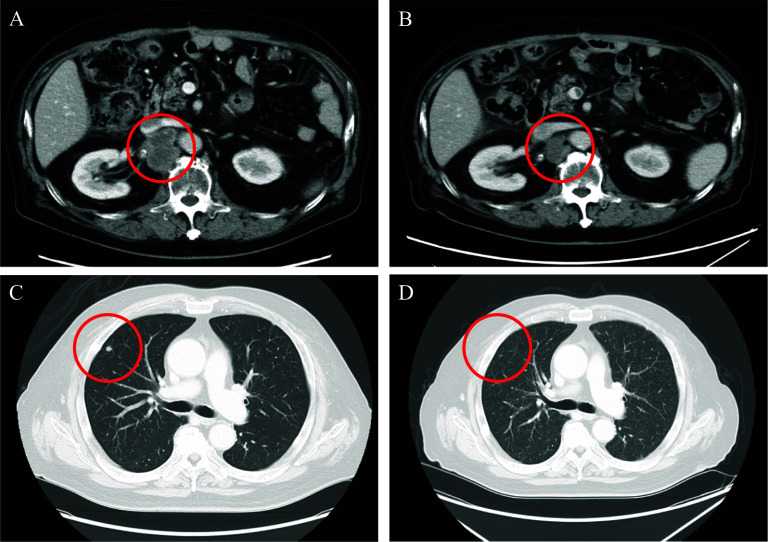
Contrast-enhanced CT findings after chemotherapy. A, B Prominent shrinkage of the enlarged metastatic para-aortic lymph node (red circle). C, D Disappearance of the lung metastases in the right upper lung lobe (red circle)
